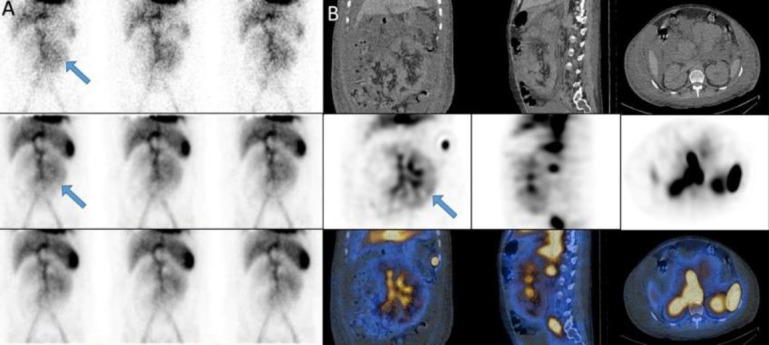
Planar and SPECT/CT images (arrows A, B) showed omentum and intestines were displaced and compressed due to ascites
